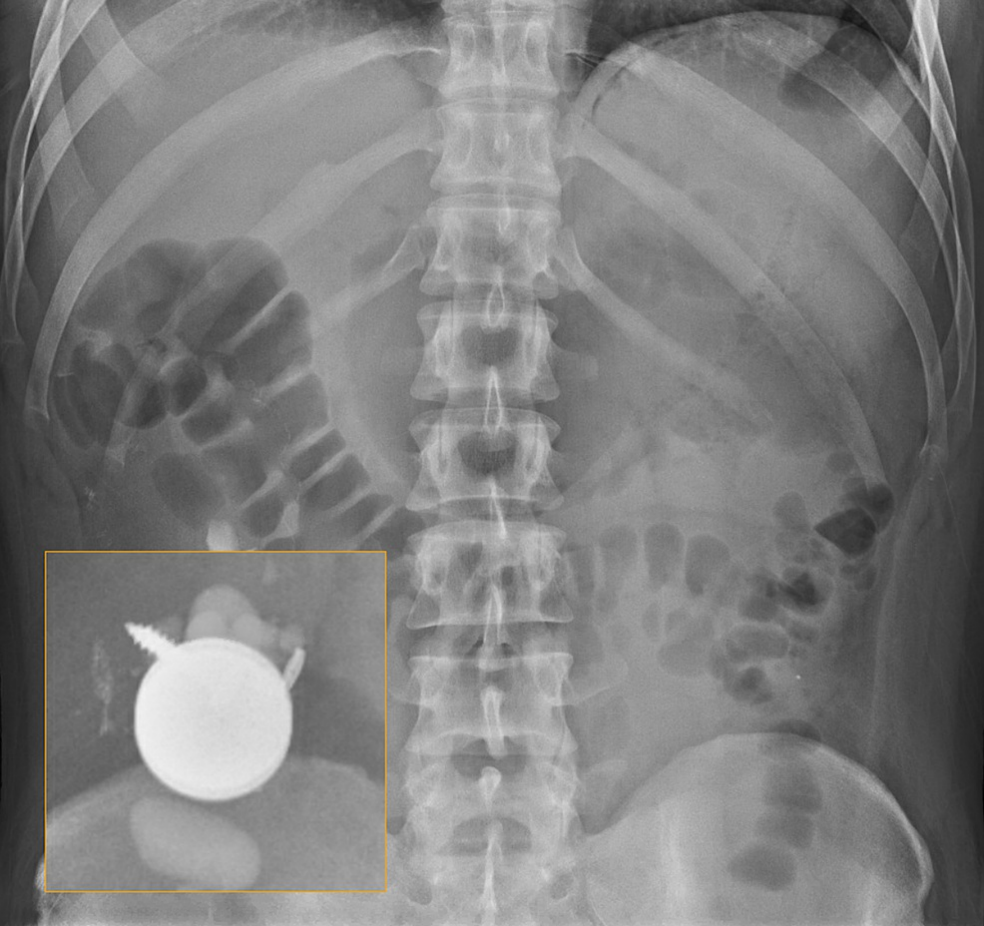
X-ray of the abdomen. Foreign objects identified in the right lower quadrant of the abdomen.
